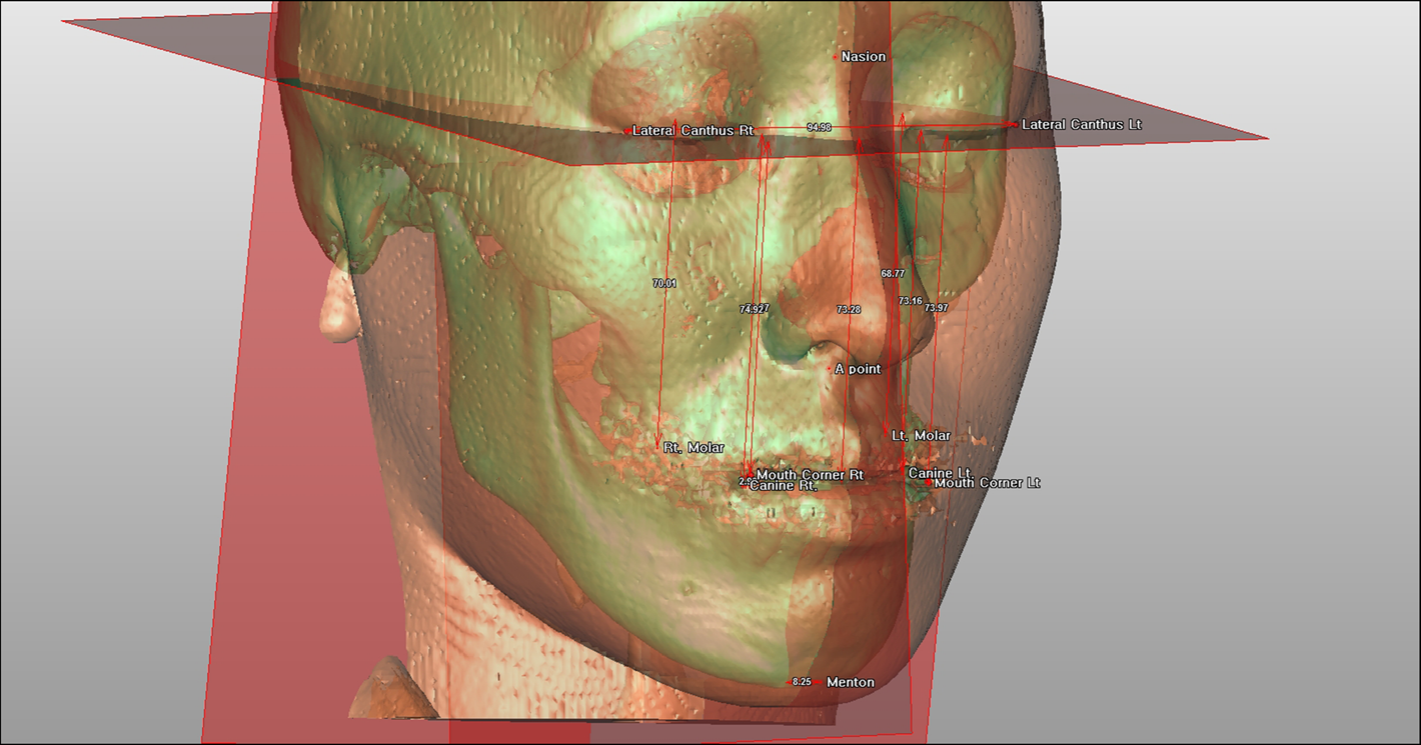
Reference planes, points, and measurements in three-dimensional CT images
